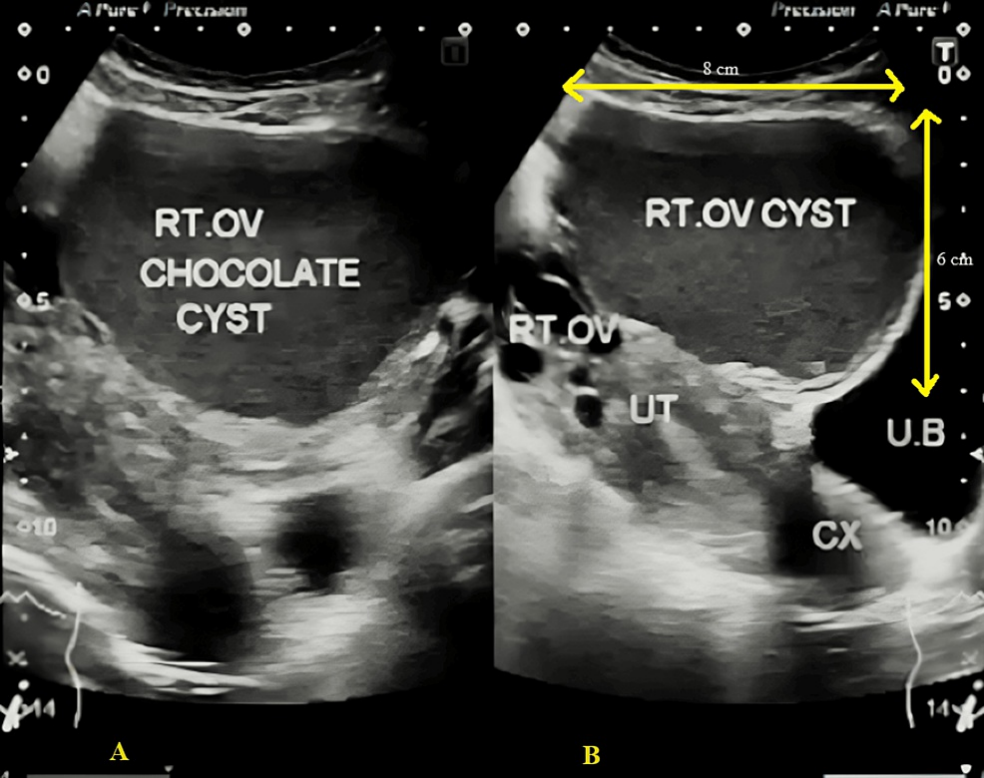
TAUS of the female patient showing chocolate cyst in right ovary (A) Axial image. (B) Sagittal image. CX, cervix; OV, ovary; RT, right; TAUS, transabdominal ultrasound; UB, urinary bladder; UT, uterus
